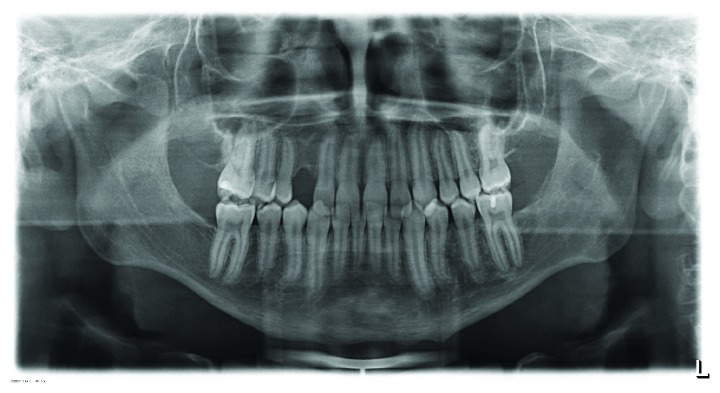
Follow-up panoramic radiograph (August 2017). Bone apposition in the former cystic cavities. No new lesions or recurrence is visible.
